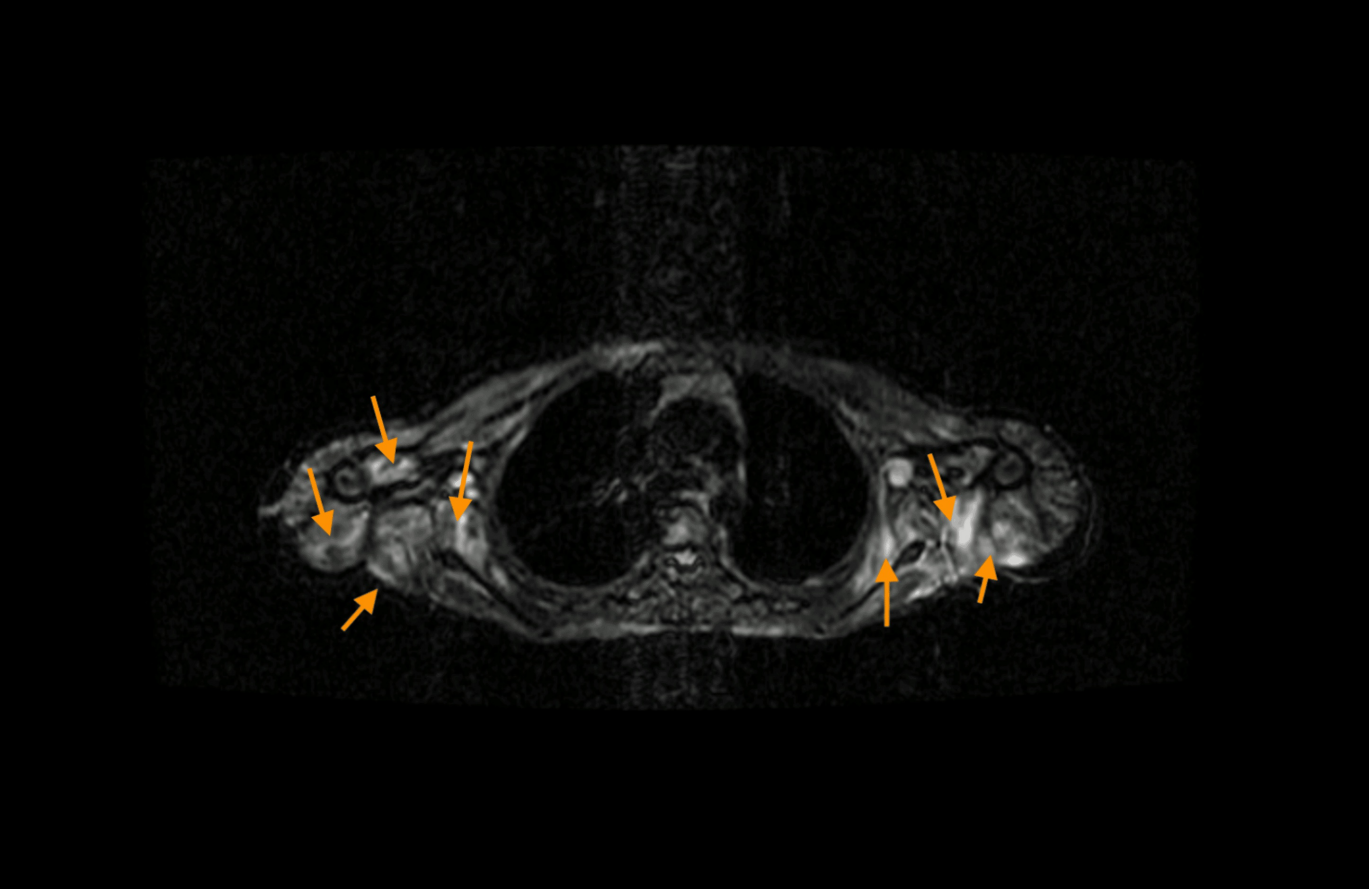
Axial STIR MRI chest image with generalized heterogeneous hyperintense signal on shoulder girdle (orange arrows) STIR - short TI inversion recovery
